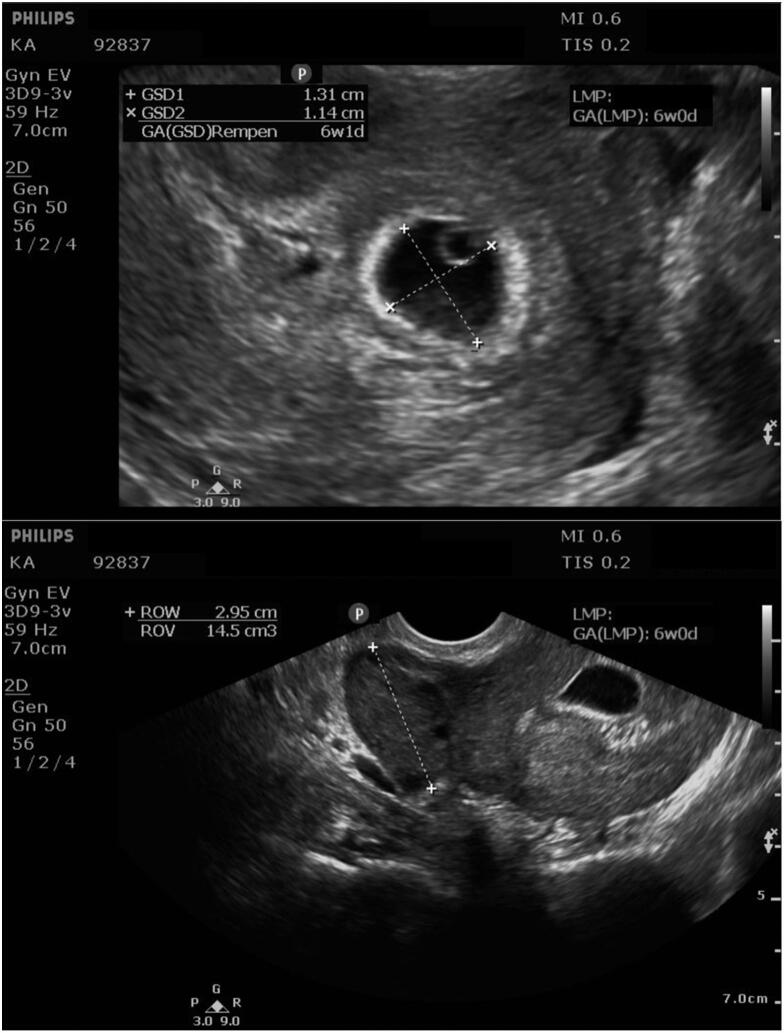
Initial transvaginal ultrasound at 6 weeks of gestation for evaluation of painless vaginal bleeding. Top: Single intrauterine gestational sac without fetal cardiac activity. Bottom: Right ovary within normal limits. *Abbreviations: Gestational Sac Diameter (GSD), Gestational Age (GA), Last Menstrual Period (LMP), Right Ovary Width (ROW), Right Ovary (ROV).*
